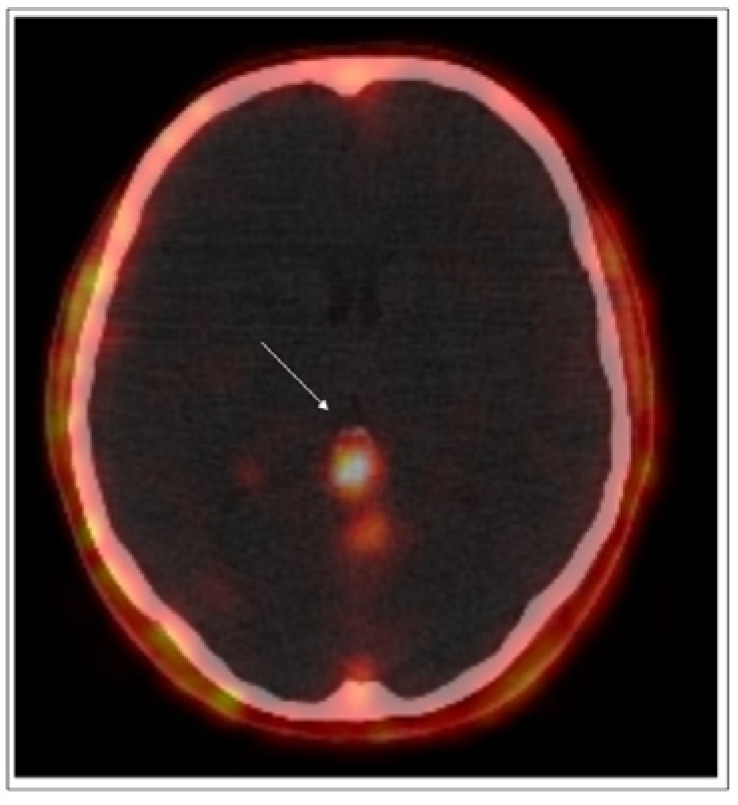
PET/CT test (68Ga-DOTA-peptide). The arrow indicates the increased somatostatin receptor expression in the pineal gland.
